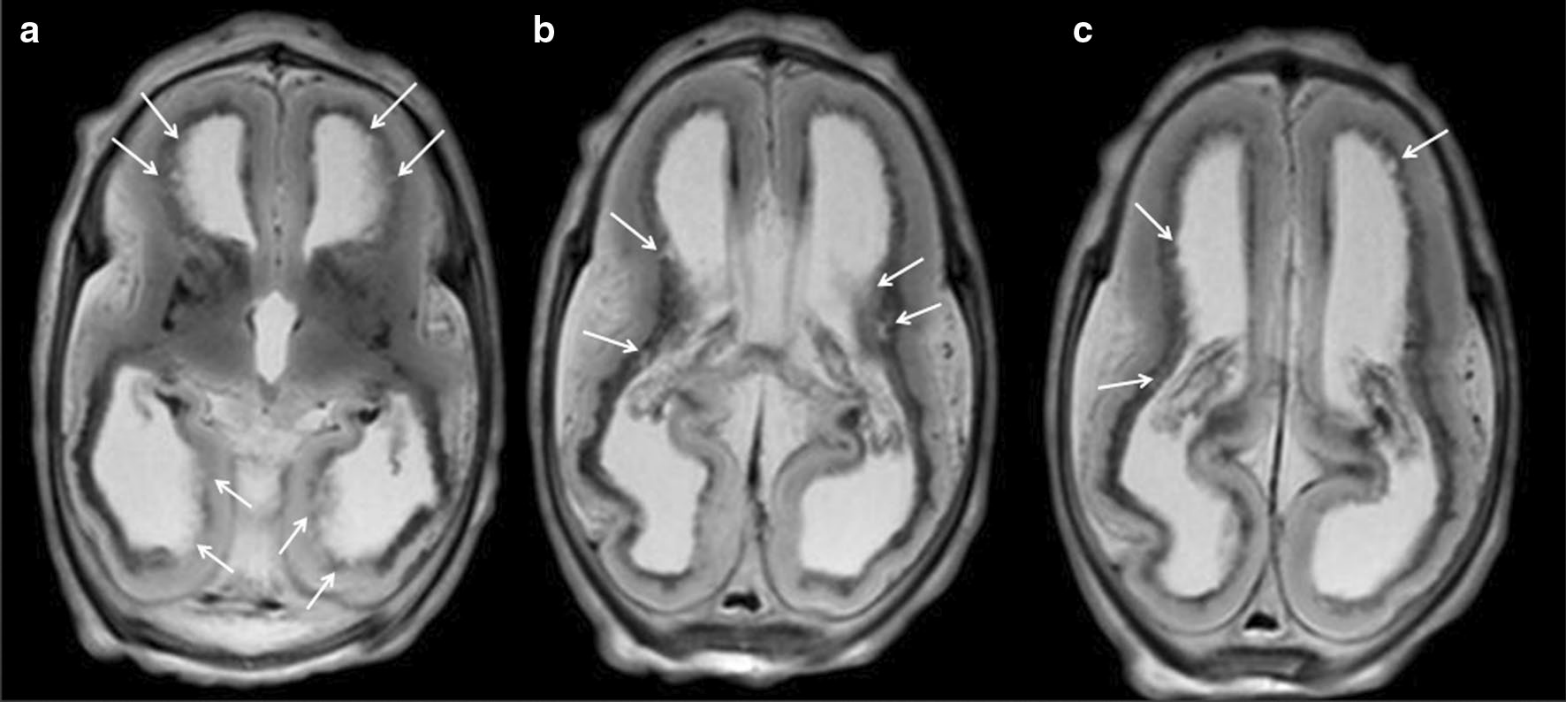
Cystic periventricular lesions in a 24-week foetus with elective termination of pregnancy due to CMV infection. a–c Axial T2W MRI showing ventricular dilatation and cystic periventricular lesions (arrows)
